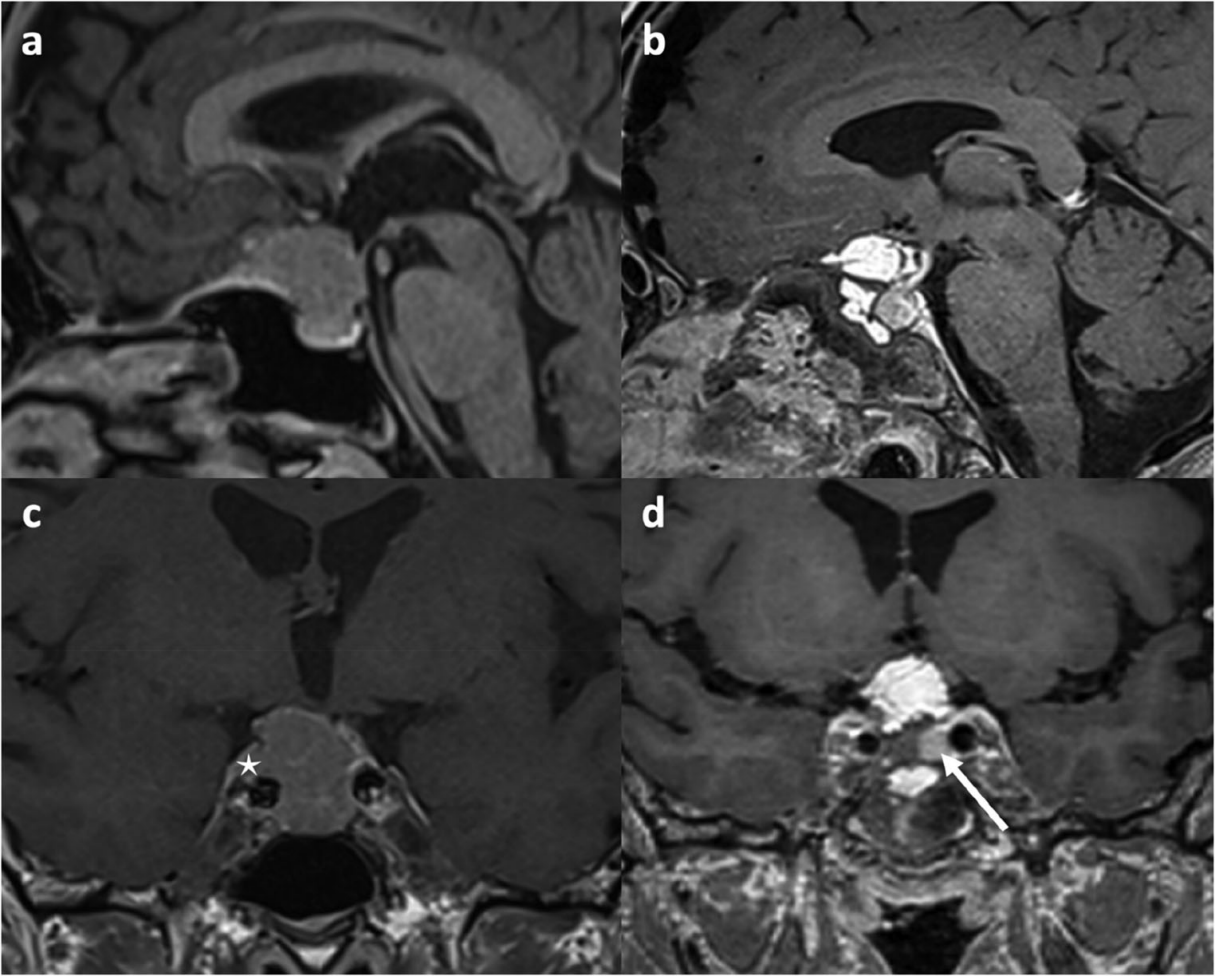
Tuberculum sellae meningioma WHO grade I (*case 2*). a–c Preoperative contrast-enhanced MRI shows a sellar and suprasellar tumor with invasion of the right cavernous sinus (asterisk). b, d Postoperative contrast-enhanced MRI demonstrating gross total resection and fat graft in the tumor cavity. The pituitary gland was displaced to the patient’s left (white arrow). Vision and visual fields improved rapidly after surgery and pituitary function was preserved
